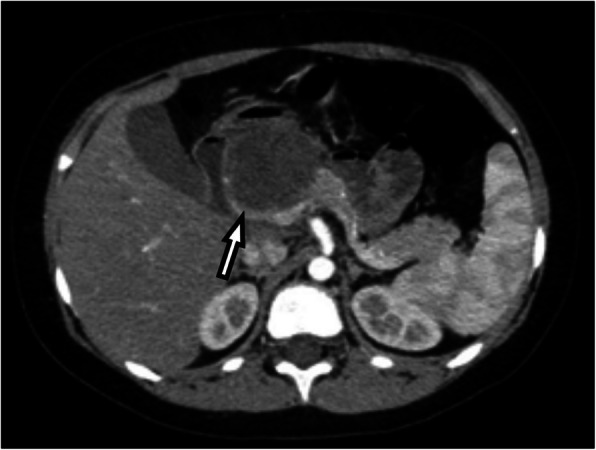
According to the CT results, an inhomogeneous hypodense formation was noted at the border of the head and the body of the pancreas, with uneven contours, 48 × 48 × 50 mm, and with contrast, there was no increase in blood flow inside the formation
